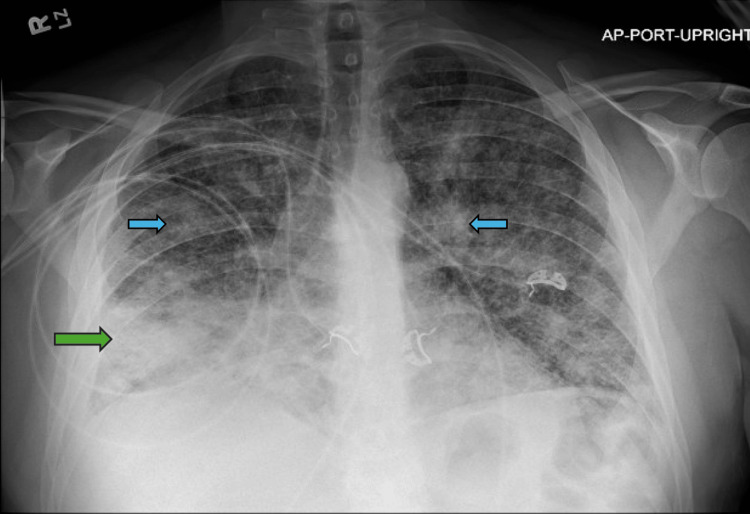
The CXR showing bilateral reticulonodular opacities (blue arrows) and right lung consolidation (green arrow) CXR: Chest X-ray
